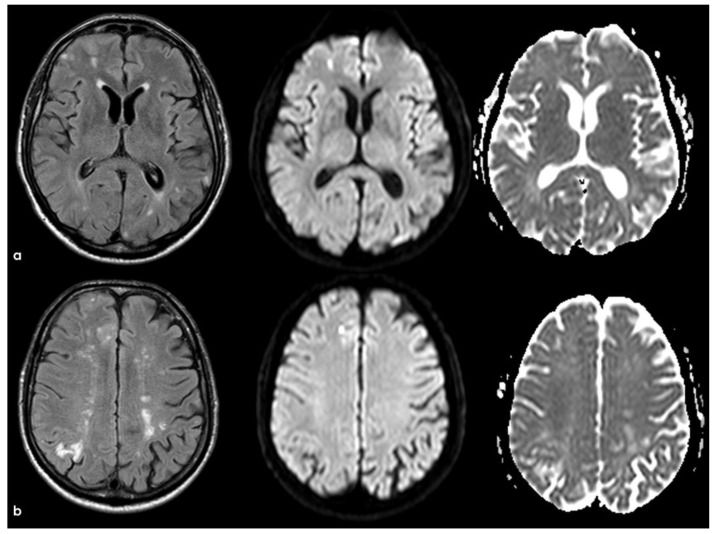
Brain MRI with axial FLAIR, DWI, and ADC slices at two different levels (panels (a) and (b)), showing two subcortical lesions in the left anterior frontal lobe, with similar signal features (hyperintense in FLAIR and DWI and hypointense on ADC map). The lesion in panel a appears less hypointense on the ADC map than the lesion in panel (b), probably reflecting a metachronous pattern.
